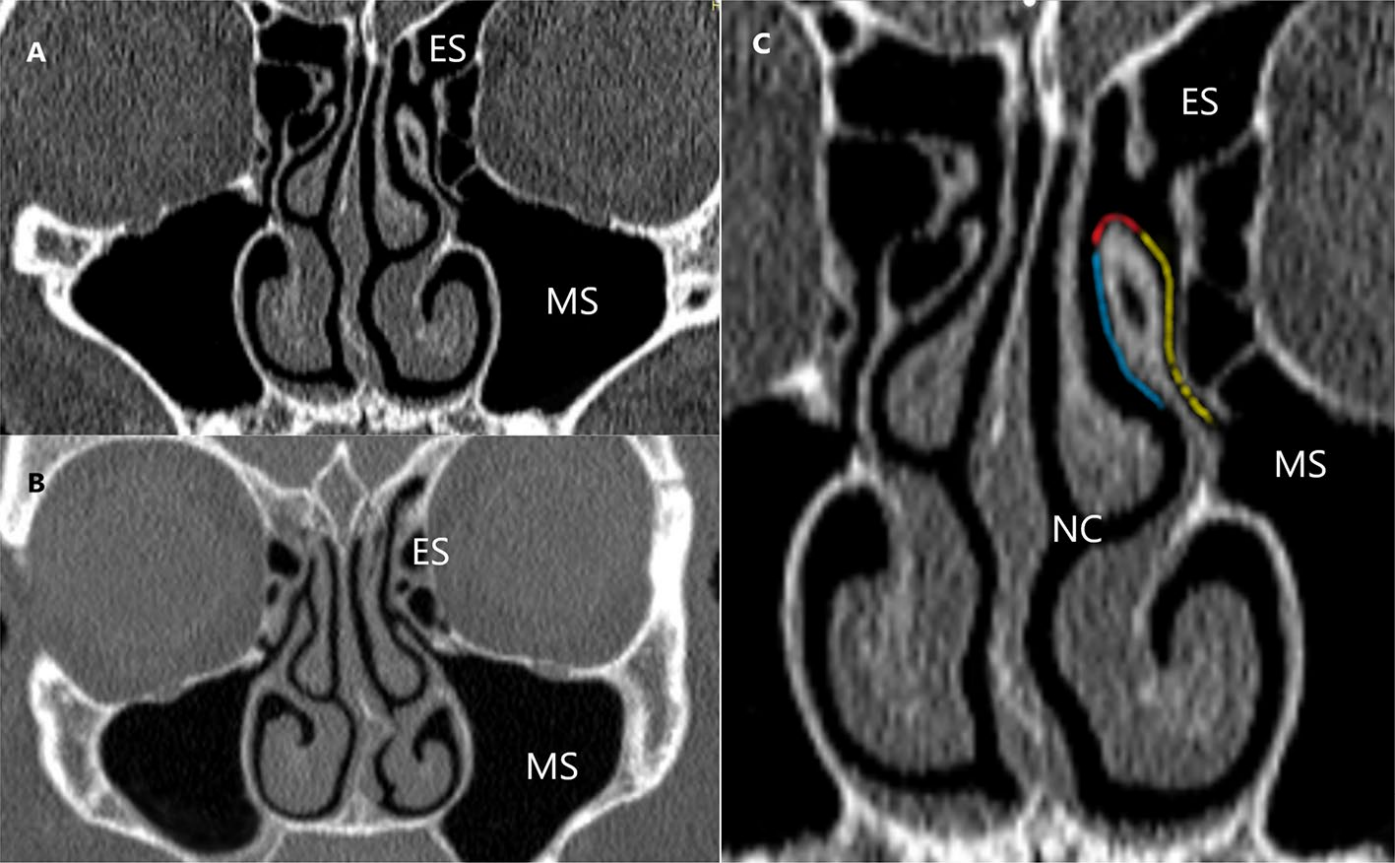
CT scans of nasal sinuses (ES—ethmoid sinus; MS—maxillar sinus; NC—nasal cavity): (A) Patient 1 (normal anatomy); (B) Patient 2 with nasal obstruction and nasal septum deviation (NSD) to the left side; (C) sinus surface of the ethmoid bone uncinate process (SUP) (yellow), the nasal surface of the ethmoid bone uncinate process (NUP) (blue); apex of the ethmoid bone uncinate process (AUP) (red).
